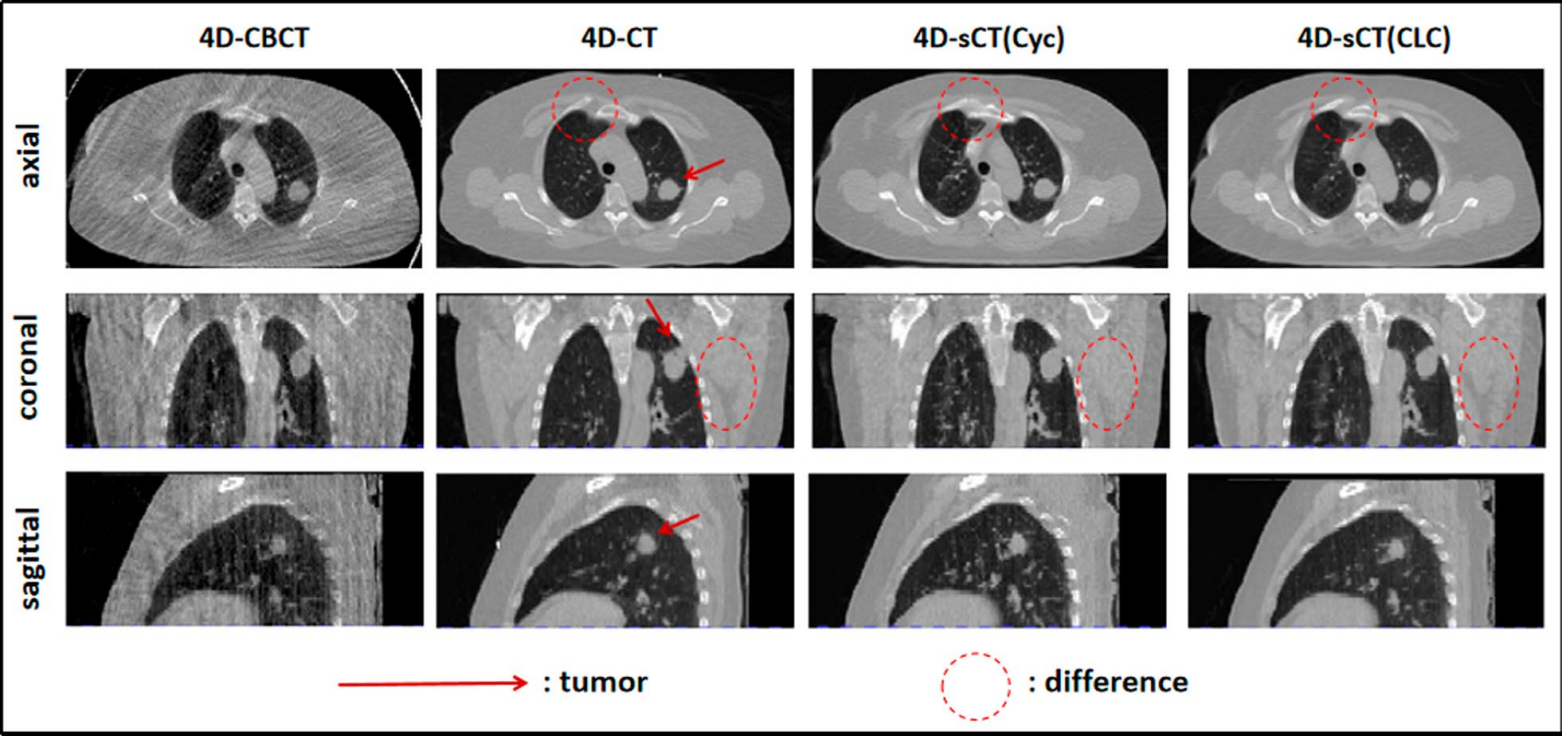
Structural Images of Patient 1 in Different Directions. The four columns represent 4D-CBCT, 4D-CT, 4D-sCT(Cyc), and 4D-sCT(CLC) images, respectively. All images are displayed at the same window width and window level
